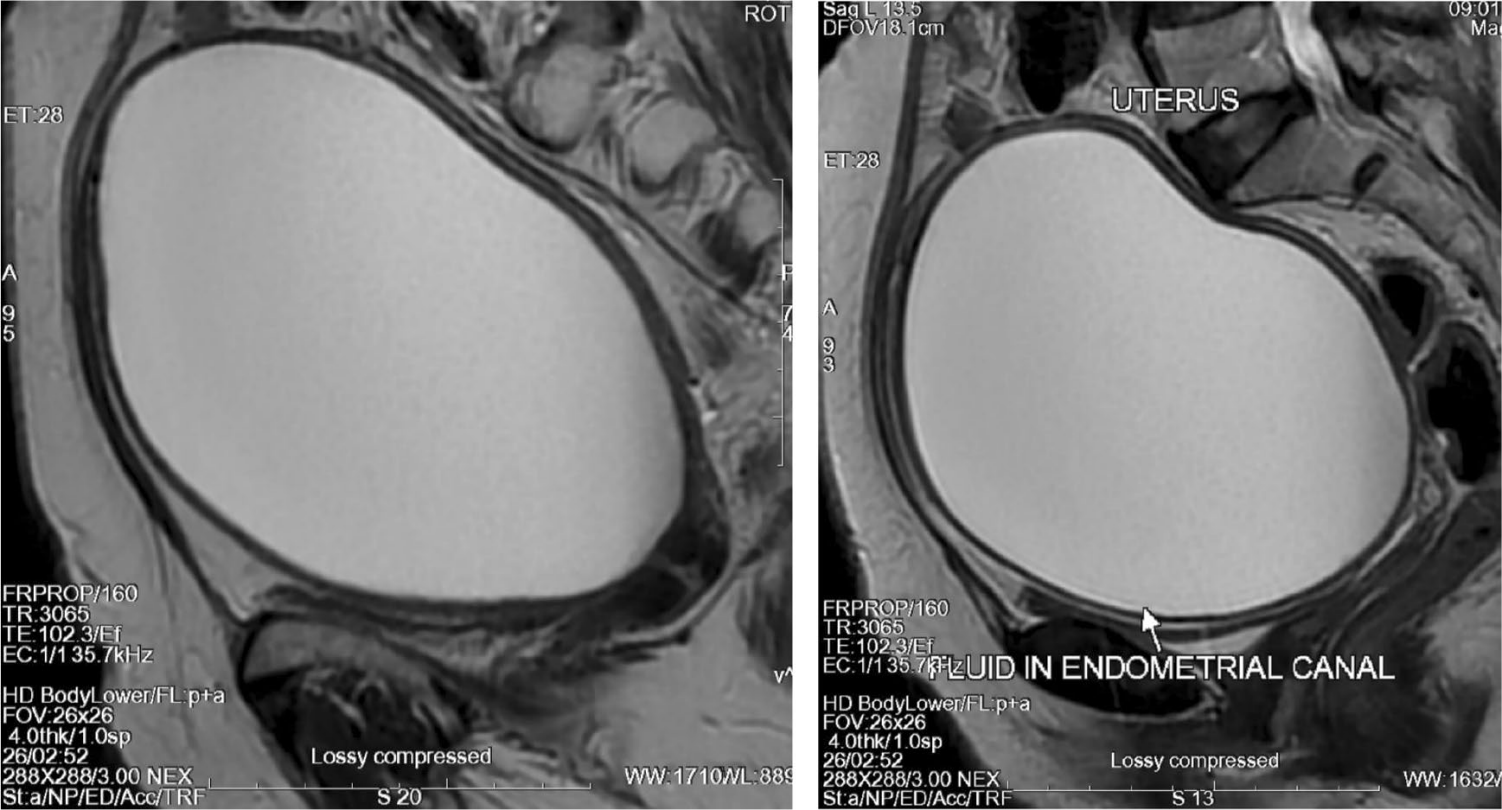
T2W MRI images showing enlarged uterus with large volume of high density fluid within endometrial cavity
